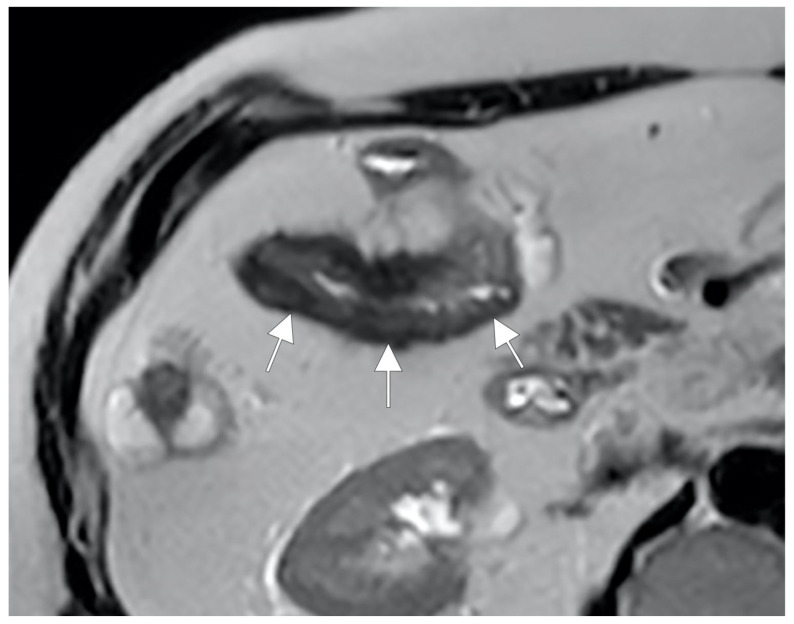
Example of marked thickening due to a benign condition. Axial T2-weighted image shows marked segmental thickening of a small bowel loop in the right abdominal quadrant (arrows), due to a benign condition (Crohn’s disease).
